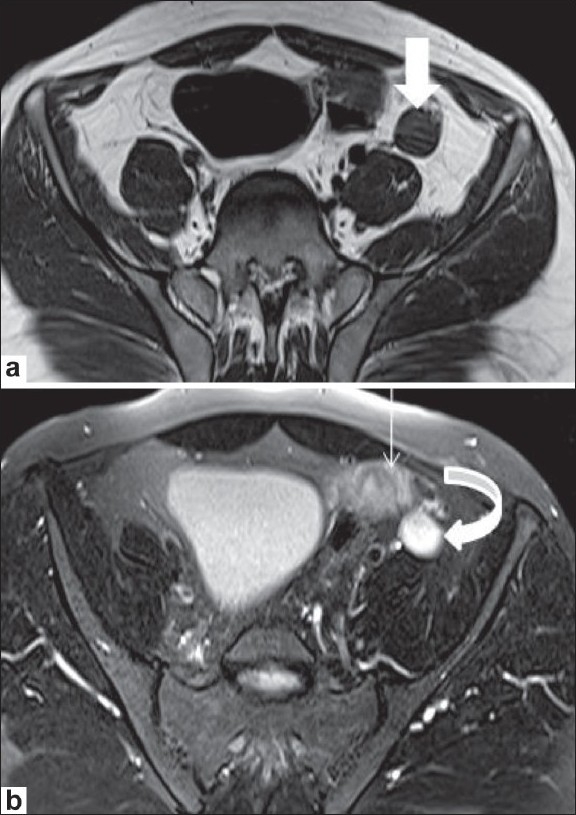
Axial T1W (a) and T2W (b) MRI images at the level of the sacroiliac joints demonstrate an oval structure with morphology and signal intensity conforming to a testis in the left inguinal region; this is isointense to muscle on the T1W (arrow in a) and hyperintense on the T2W (curved arrow in b) images. The fallopian tube (thin arrow in b) is seen along with the testis
